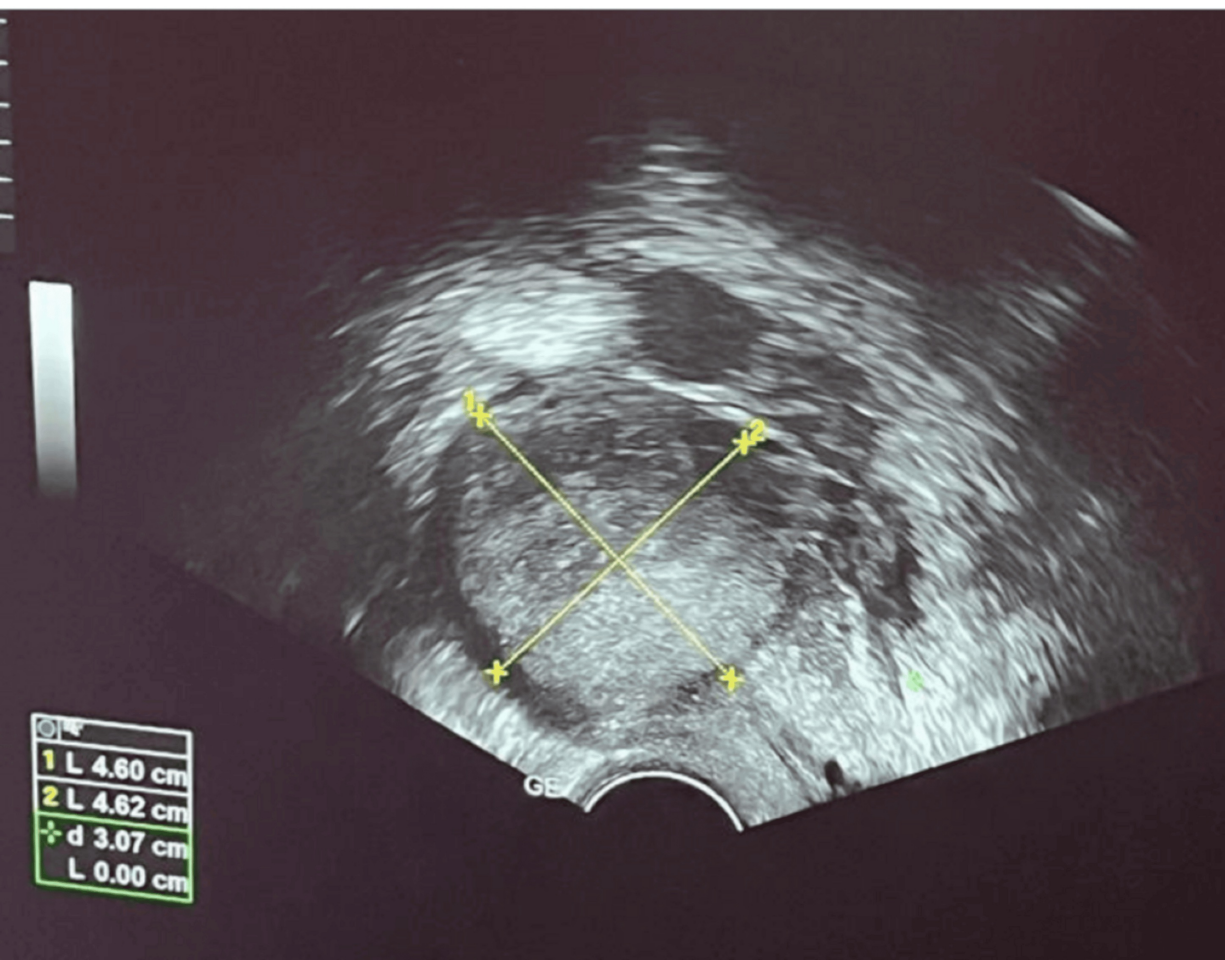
Pelvic ultrasound demonstrating FIGO type 2-5 submucosal fibroid measuring 4.6 × 4.6 cm in the anterior fundal region. FIGO, International Federation of Gynecology and Obstetrics
